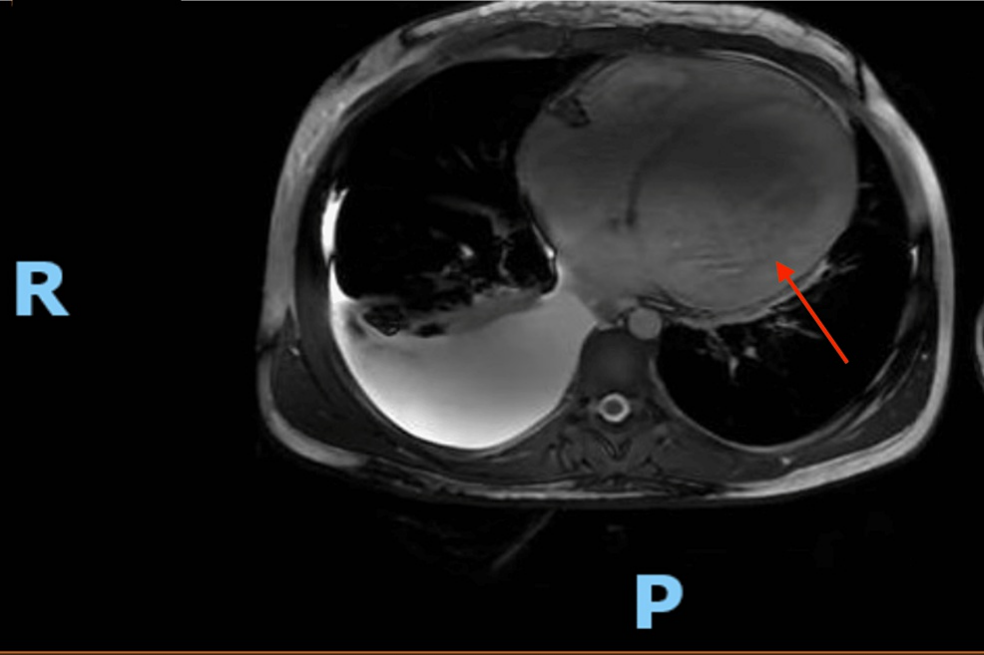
MRI of methamphetamine-induced cardiomyopathy This MRI shows the extensively dilated ventricles in this case. The left ventricle (marked by a red arrow) is remarkably dilated. The study was taken during an episode of noteworthy acute decompensated CHF and demonstrates the magnitude of the pathology. Massive right-sided posterior pleural effusion is noteworthy regarding the crescent shaped fluid collection in the right/posterior chest cavity (marked as R for right side and P for posterior).
